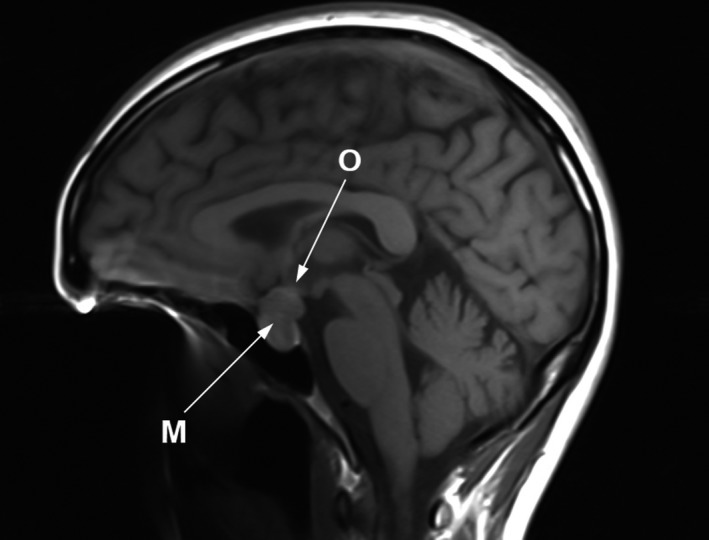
T1‐MRI Pituitary sagittal scan at 2 wk postpartum: macroadenoma (M) with significant compression of optic chiasm (O)
